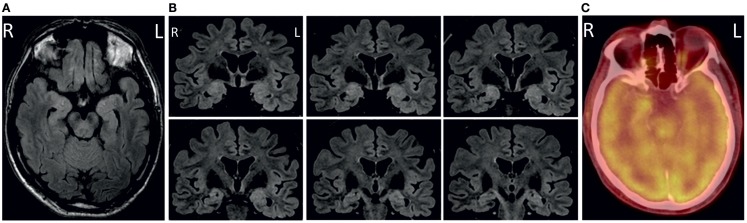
Axial (A) and coronal (B) fluid-attenuated inversion recovery (FLAIR) images showing bilateral volume and signal increase in amygdala and anterior hippocampus. Correspondingly, cerebral fluor-18-deoxyglucose-positron emission tomography/computed tomography (FDG-PET/-CT) revealed hypermetabolism of the right anterior mesial temporal lobe (C).
